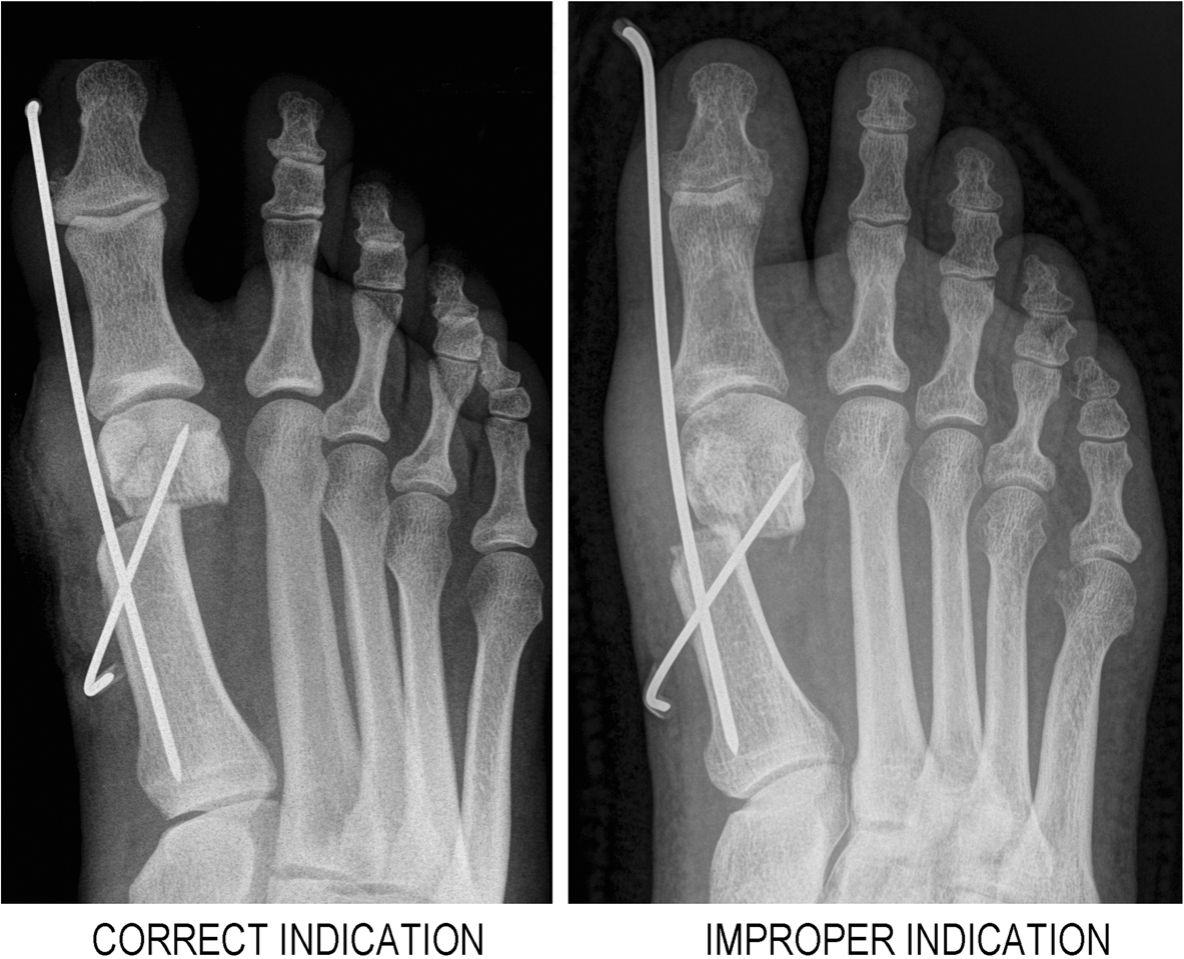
Clinical examples of correct and improper MIDMO indication. *Left* part of the figure shows correct indication for MIDMO with sufficient postoperative contact between fragments and optimal postoperative correction. *Right* part of the figure shows MIDMO with improper indication because of too large bunion exostosis (5.5 mm) for the given subcapital neck width (13 mm). Geometrical analysis of preoperative radiograph predicts only 4 mm of postoperative contact, and this was even further reduced to 3 mm due to intraoperative plantar displacement of the distal fragment, finally resulting in pseudoarthrosis
